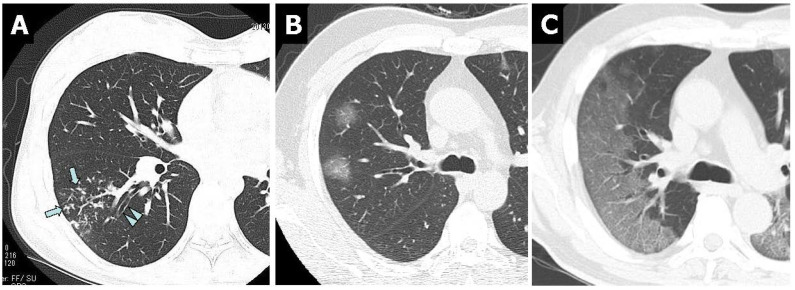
Non-contrast-enhanced thin-section axial images of the lungs in patients with *M. pneumoniae* pneumonia (A) and COVID-19 pneumonia (B,C). (A) Chest CT scan of a 46-year-old female showed nodules (tree-in-bud, arrows) and bronchial wall thickening (arrowheads). (B) Chest CT scan of a 46-year-old man showed bilateral and multifocal rounded GGO. (C) Chest CT in a 56-year-old man showed bilateral and peripheral GGO with superimposed interlobular septal thickening and crazy-paving appearance.
